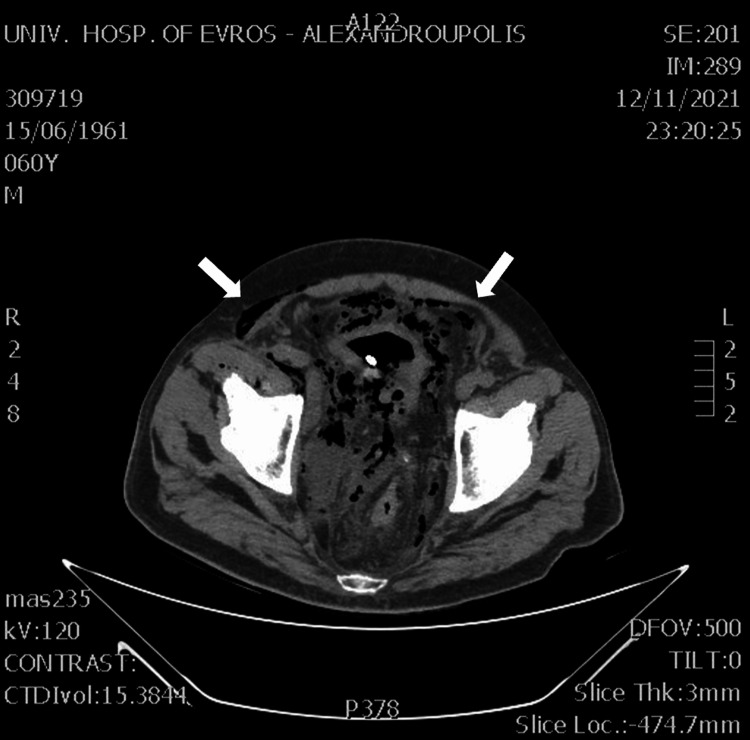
CT examination of the abdomen revealed fat stranding and free air around the bladder (white arrows). CT: computed tomography.
